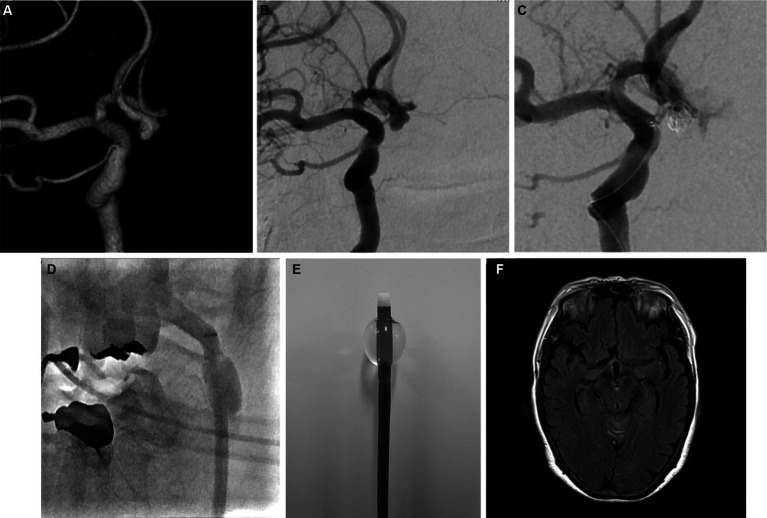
Representative pre- and postprocedural images. (A) Three-dimensional and (B) digital subtraction angiography (DSA) images showing an unruptured anterior communicating aneurysm. (C) DSA image showing active contrast extravasation due to aneurysm perforation. (D) Angiography image showing proximal flow control with balloon guiding catheter during intraprocedural rupture. (E) Inflated balloon guiding catheter (Flowgate2; Stryker, United States). (F) Magnetic resonance image (axial view) showing no subarachnoid bleeding in the basal cistern 1 day after perforation.
